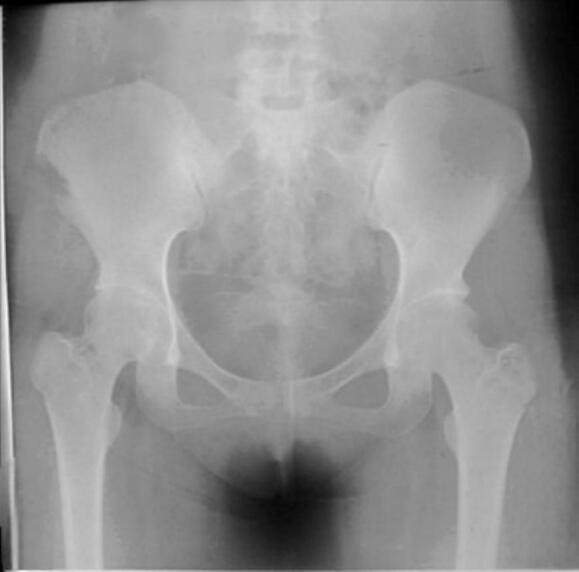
Postoperative X-ray of the hip for patient II post bilateral core decompression.
